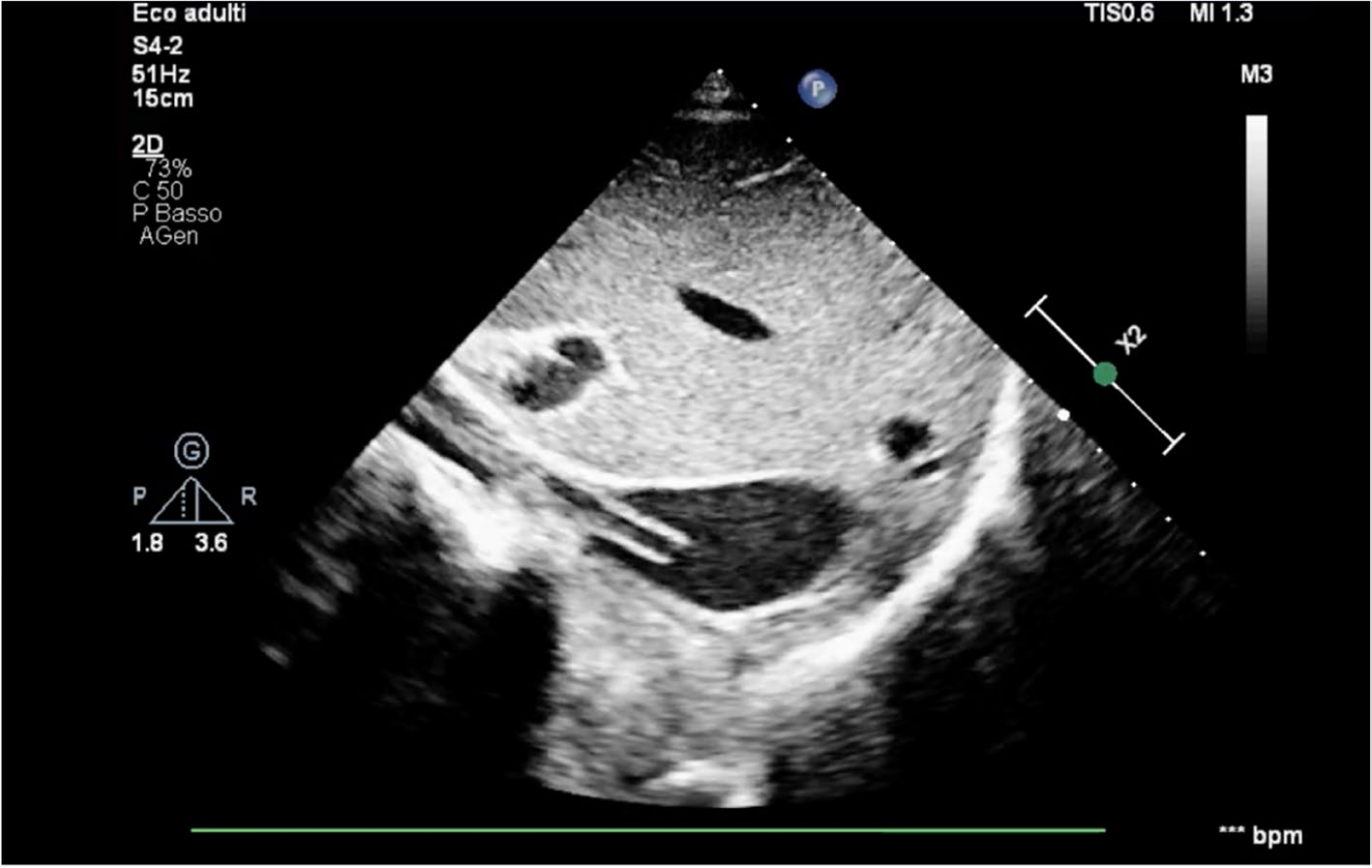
Ultrasound assessment of the inferior vena cava at its confluence with the right atrium: longitudinal view of a fibroblastic sheath
